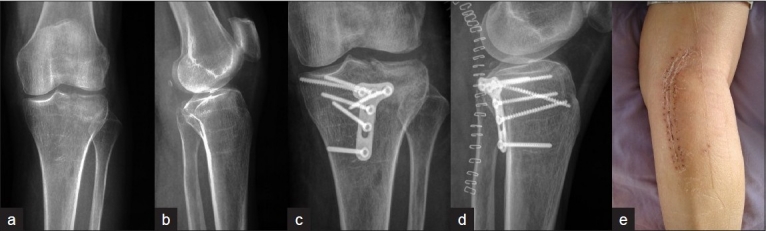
Anteroposterior (a) and lateral (b) radiographs showing the fragment of tibial plateau fracture locating posterolateral in Patient 5. Postoperative anteroposterior (c) and lateral (d) radiographs show satisfactory reduction and fixation through a direct PL approach. Clinical photograph of knee and leg, shows scar of PL approach (e). PL= Posterolateral
